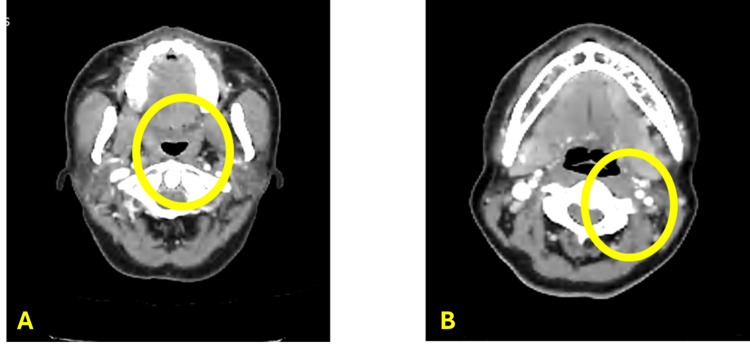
CT findings after the induction chemotherapy A.The image on the left side revealed complete interval shrinkage of the enhanced mass in the left palatine tonsil. B. The image on the right side also revealed complete shrinkage at the level II neck region CT: computed tomography
